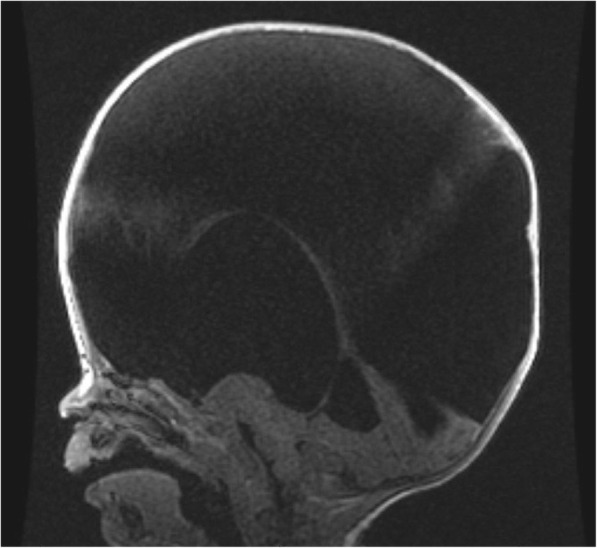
MRI sagittal view with brain parenchyma in posterior fossa, cerebral falx, thalamus, brainstem, and cerebellum visualized
